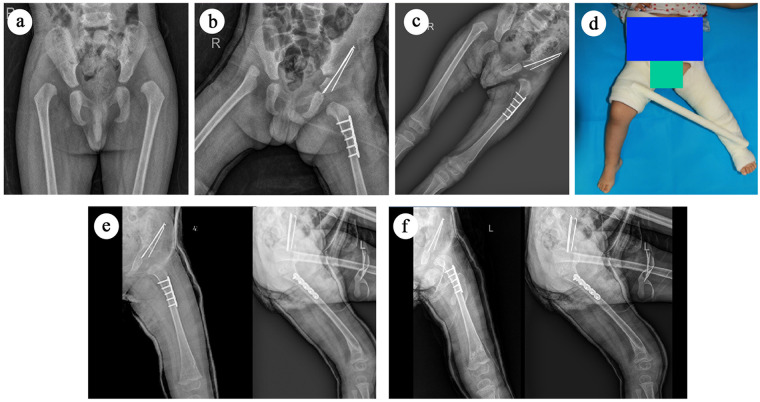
Bilateral DDH in a 2-year-old girl. (a) Radiograph showing bilateral DDH. (b) Salter and left proximal femoral osteotomy. (c) A mildly displaced fracture of the distal femur was found 1 month after PFO. (d) Appearance after early spica cast. (e) Radiograph obtained the day after early spica casting. (f) Two-month radiograph after spica cast fixation. DDH, developmental dysplasia of the hip; PFO, proximal femoral osteotomy.
